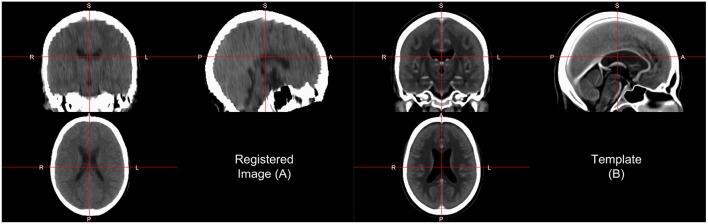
Image registration result. Here we displayed the scan (A) registered to a CT template (B) from Rorden et al. (2012). The registration by first doing an affine registration, followed by symmetric normalization (SyN), a non-linear registration implemented in ANTsR. The registration was done with the skull on the image and the template. We see areas of the image that align generally well, but may not be perfect.
